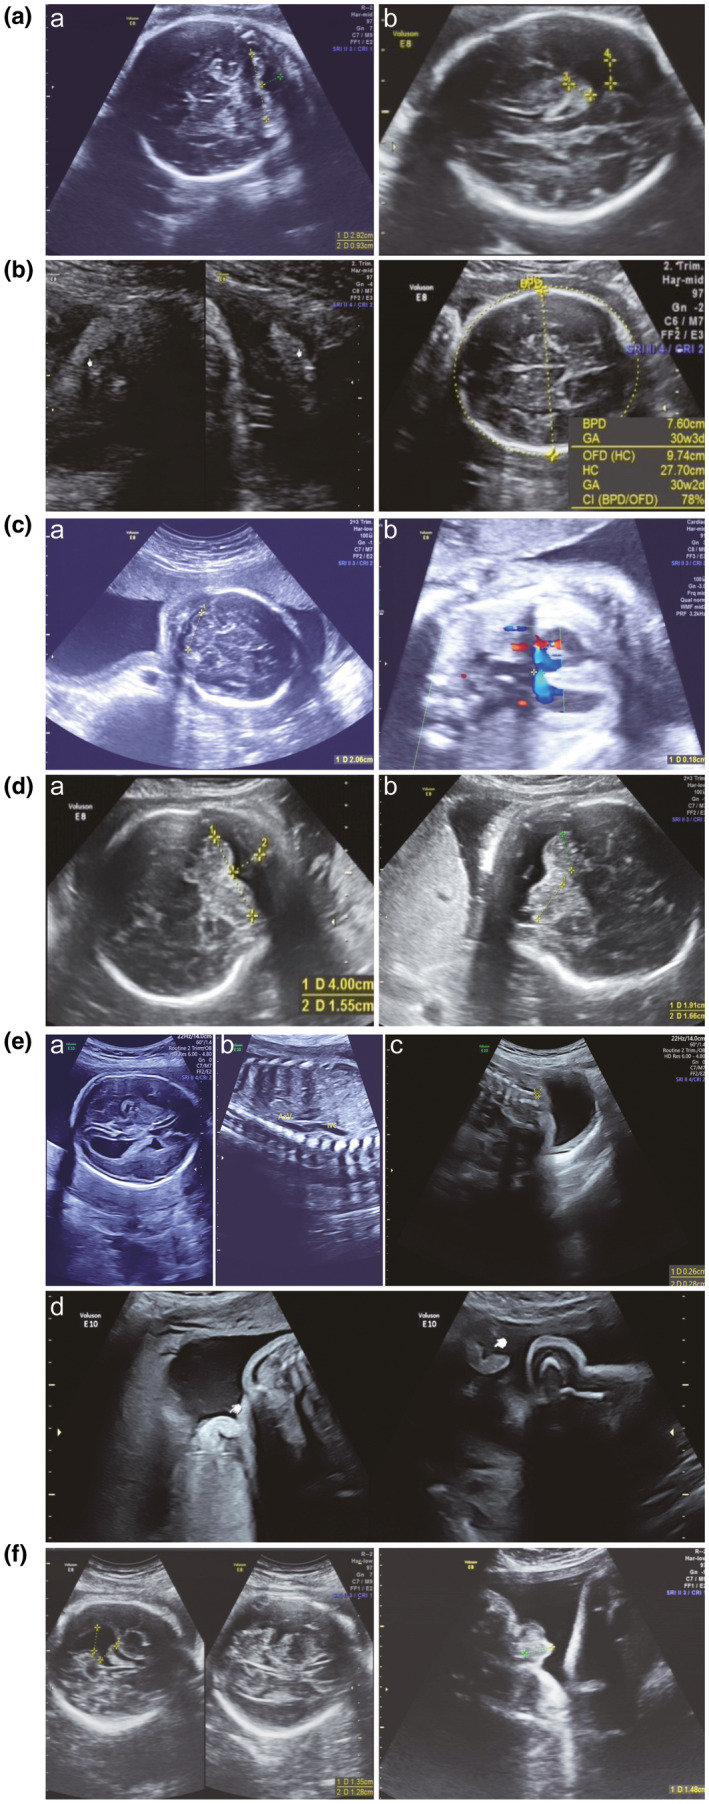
Images of ultrasound test on fetuses. A. Images of ultrasound test on fetus 2 at 30 weeks’ gestation. (a) Ultrasonographic image showing measurement of transverse cerebellar diameter (TCD). The TCD of this fetus was 2.92 cm. The normal value at 30 weeks’ gestation is 3.86 ± 0.34. The value of posterior fossa pool is 0.93 cm that is higher than the normal range (<0.80 cm). (b) Ultrasonographic image showing measurement of the left lateral ventricle. The highest value is 1.06 cm that is higher than the normal range (<0.80 cm). B. Images of ultrasound test on fetus 3 at 36 weeks’ gestation. (a) Ultrasonographic image showing that the lung tissue was scarce, suggesting lung dysplasia. (b) Ultrasonographic image showing measurement of biparietal diameter (BPD) and head circumference (HC). The value of BPD (7.6 cm) and HC (27.70 cm) represent 30 weeks’ gestation, suggesting an intrauterine growth retardation of fetus 3. C. Images of ultrasound test on fetus 4 at 24 weeks’ gestation. (a) Ultrasonographic image showing measurement of TCD. The TCD of fetus 4 was 2.06 cm. Normal value at 24 weeks’ gestation is 2.85 ± 0.17 cm. (b) Ultrasonographic image showing transventricular septal blood flow signal, suggesting ventricular septal defect. The ventricular septal defect sized 0.3 cm. D. Images of ultrasound test on fetus 5 at 35 weeks’ gestation. (a) Ultrasonographic image showing the TCD of this fetus was 4.00 cm. The normal value at 35 weeks’ gestation is 4.29 ± 0.26. The value of posterior fossa pool was 1.55 cm that is higher than the normal range (<0.80 cm). (b) Ultrasonographic image showing measurement of left and right cerebellar hemisphere, suggesting left cerebellar hemispheric dysplasia. E. Images of ultrasound test on fetus 7 at 26 weeks’ gestation. (a) Ultrasonographic image showing measurement of the left lateral ventricle. (b) Ultrasonographic image showing absence of inferior vena cava. (c) Ultrasonographic image showing neural tube defects. (d) Ultrasonographic image showing hypospadias. F. Images of ultrasound test on fetus 10 at 32 weeks’ gestation. (a) Ultrasonographic image showing measurement of the left lateral ventricle. The result of measurement was 1.28–1.35 cm. (b) Ultrasound image showing the coronal view of the external genitalia, suggesting hypospadias
